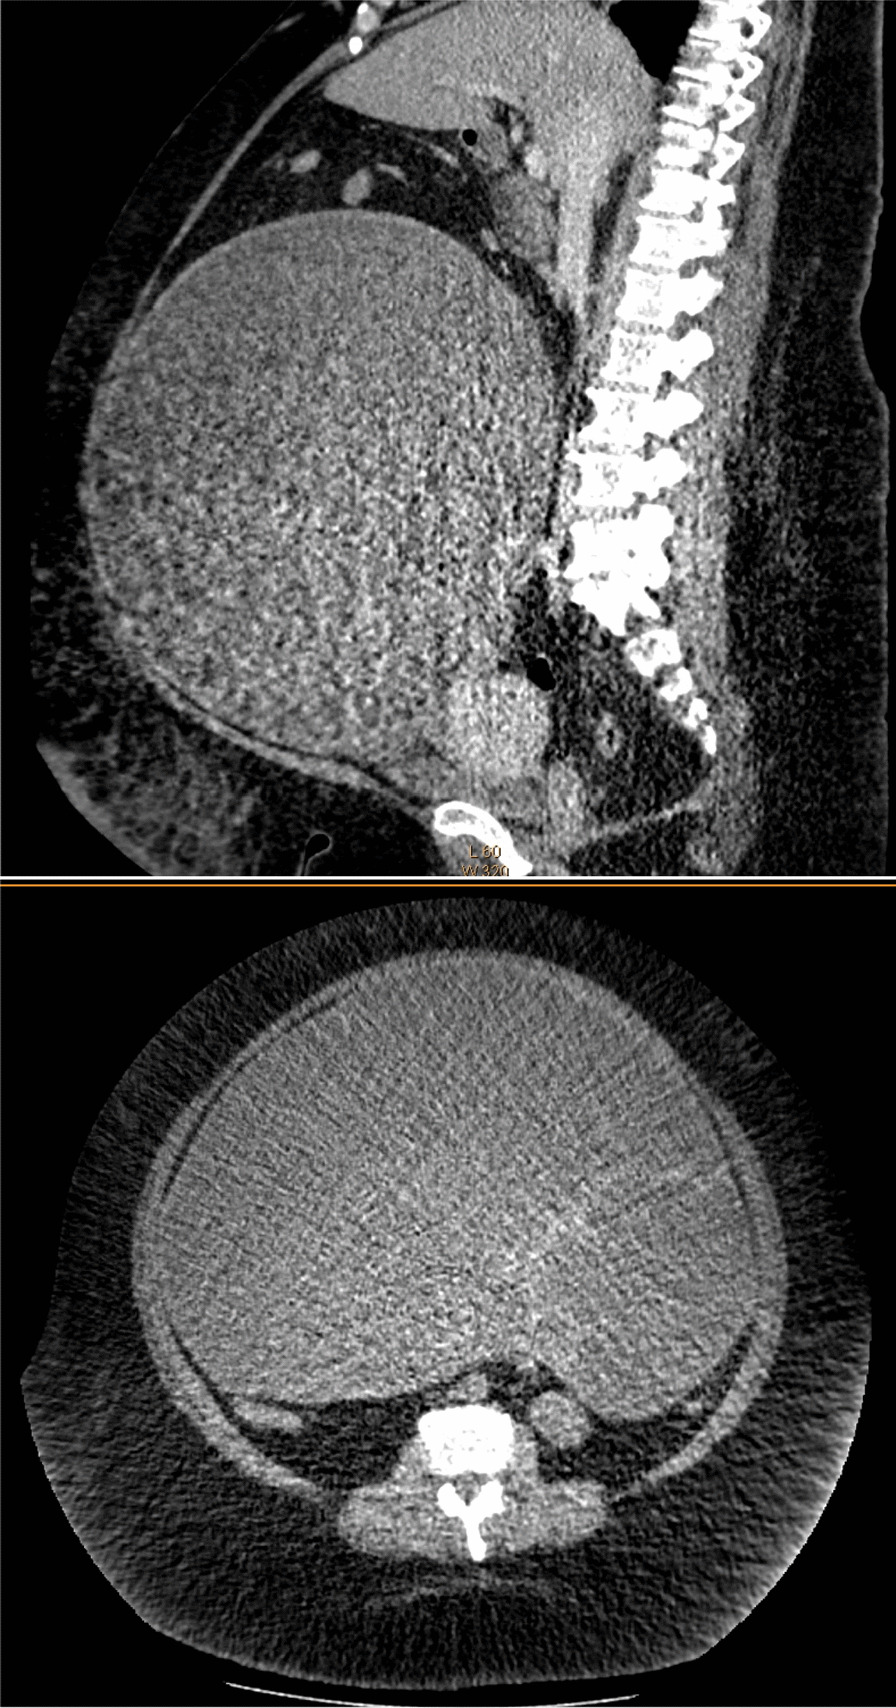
Preoperative abdominal CT shows a giant cystic tumor. Sagittal plane and transverse plane
